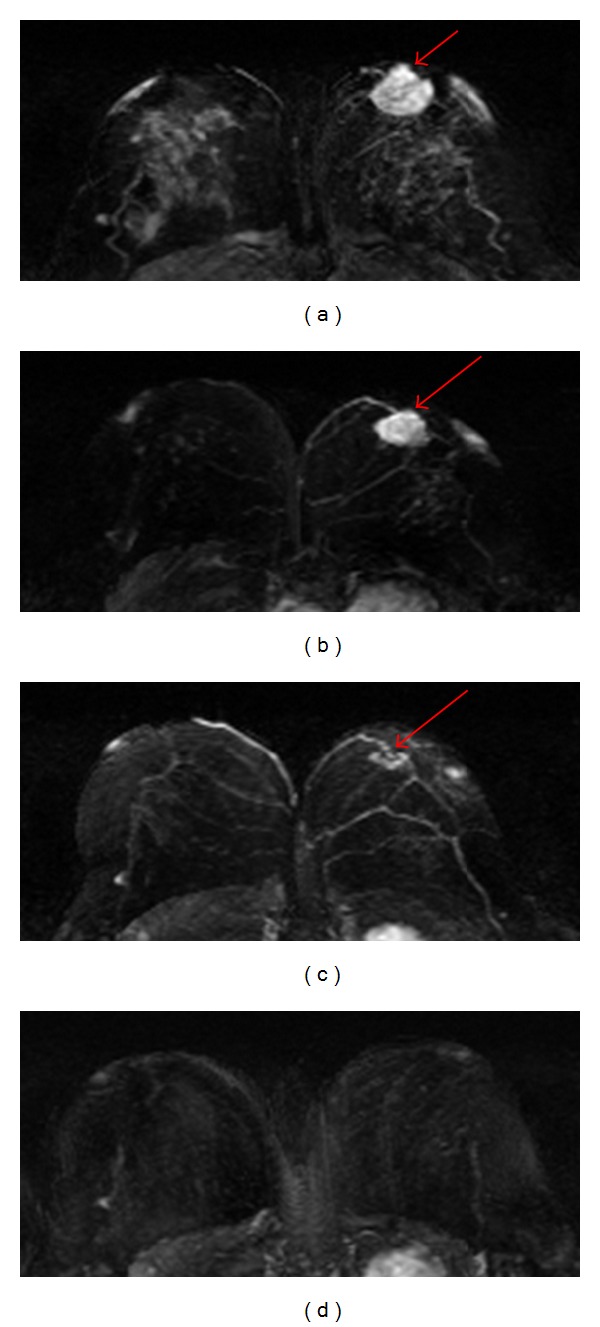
A 41-year-old patient with a mass lesion (invasive ductal cancer) in the left breast. From (a) to (d), the maximum intensity projection (MIP) images of pretreatment, F/U-1, F/U-2, and F/U-3 MRI are shown. The tumor size is 4.0 cm before treatment, which shrinks down to 2.7 cm in F/U-1 (32% reduction), 1.2 cm in F/U-2, and reaches a complete response in F/U-3 after completing NAC. This patient is confirmed as pCR in post-NAC pathological examination. The total choline concentration measured by MRS is [tCho] = 2.33 ± 0.54 mmol/kg before therapy, which decreases to 1.15 ± 0.25 mmol/kg in F/U-1, showing 51% reduction. Tumor size is too small in F/U-2 and F/U-3 for MRS measurements. For this pCR case, the tumor size reduction is 32% at F/U-1, and [tCho] reduction is greater at 51%.
